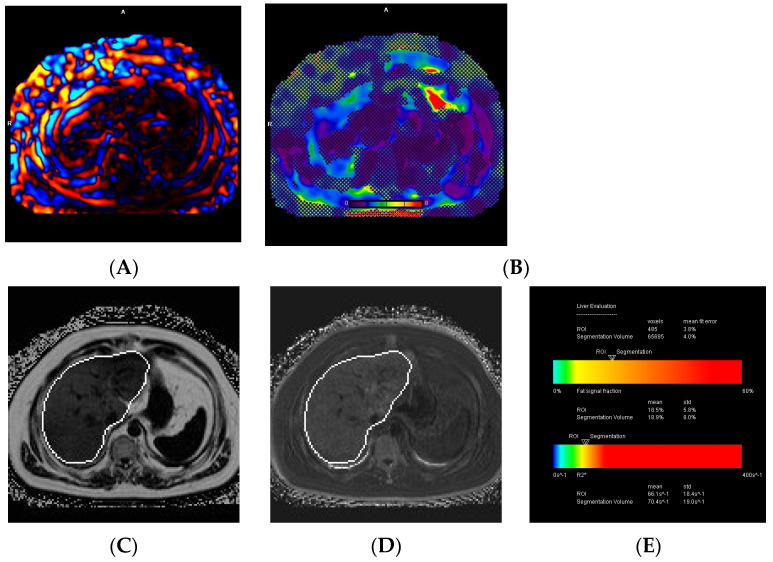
A 60-year-old male with MASLD. Wave (A) and elastogram (B) MRE images show a normal or inflamed liver with a stiffness of 2.5 kPa. MRI-PDFF map (C), R2* map (D), and the liver evaluation table (E) demonstrate moderate steatosis (18.9%) and borderline iron overload (R2* of 70.4 s^−1^ and a LIC of 2.3 mg/g) based on segmentation.
